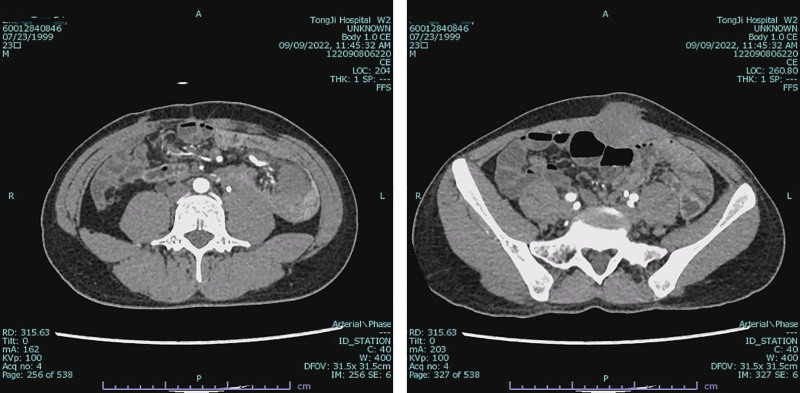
Preoperative CT image of patient in Case 2. CT = computed tomography.
